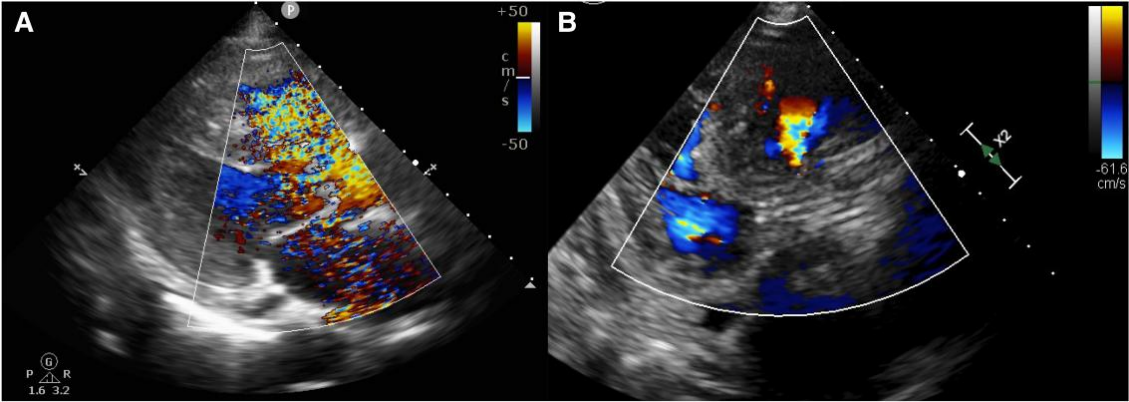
(*A*) Trans-thoracic echocardiogram before trans-catheter aortic valve replacement showing left-to-right shunt. (*B*) Trans-catheter aortic valve replacement showing decreased left-to-right shunt and good placement of the implanted valve.
